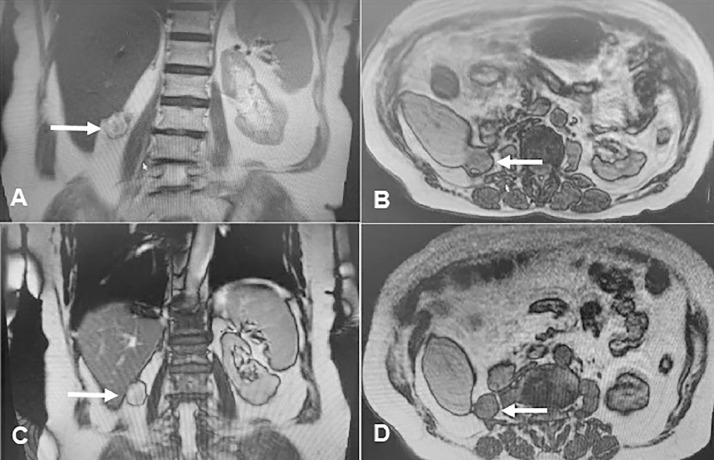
Patient's Abdominopelvic MRI after the surgery. (A and B) 8 month after the surgery. The arrows demonstrate the presence of Surgicel at the surgical site. (C and D) 2 years after the surgery. The arrows indicate the presence of Surgicel at the surgical site, with a smaller size compared to the initial imaging.
